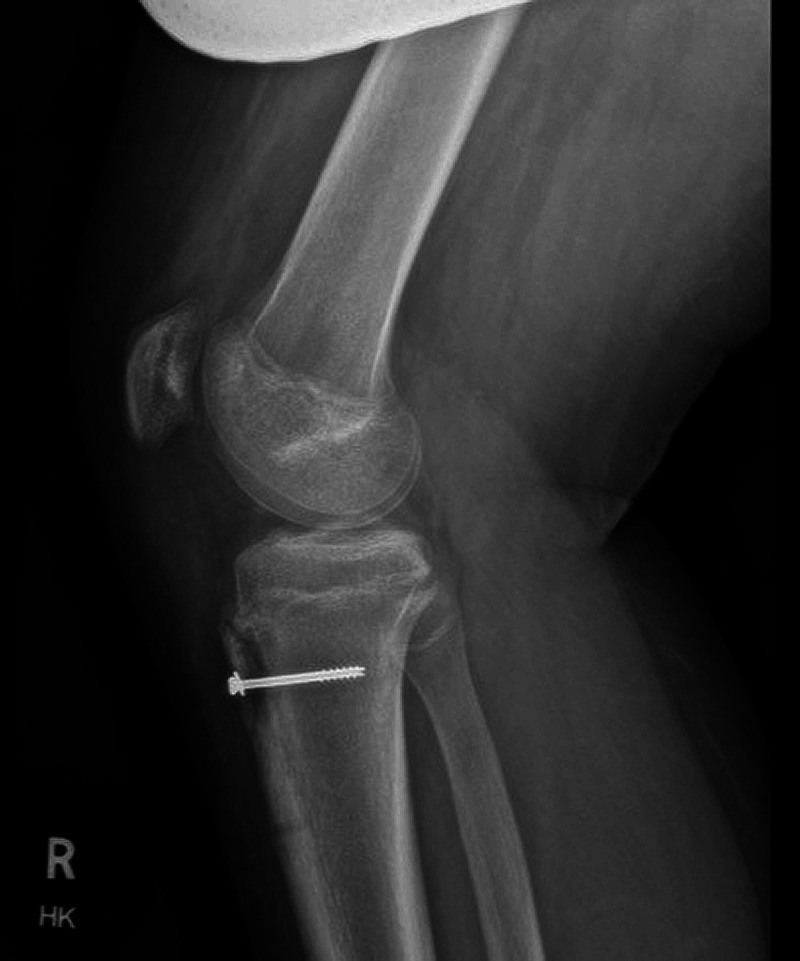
Postoperative lateral radiograph demonstrates an isolated screw securing the bone fragment to the tibia.
